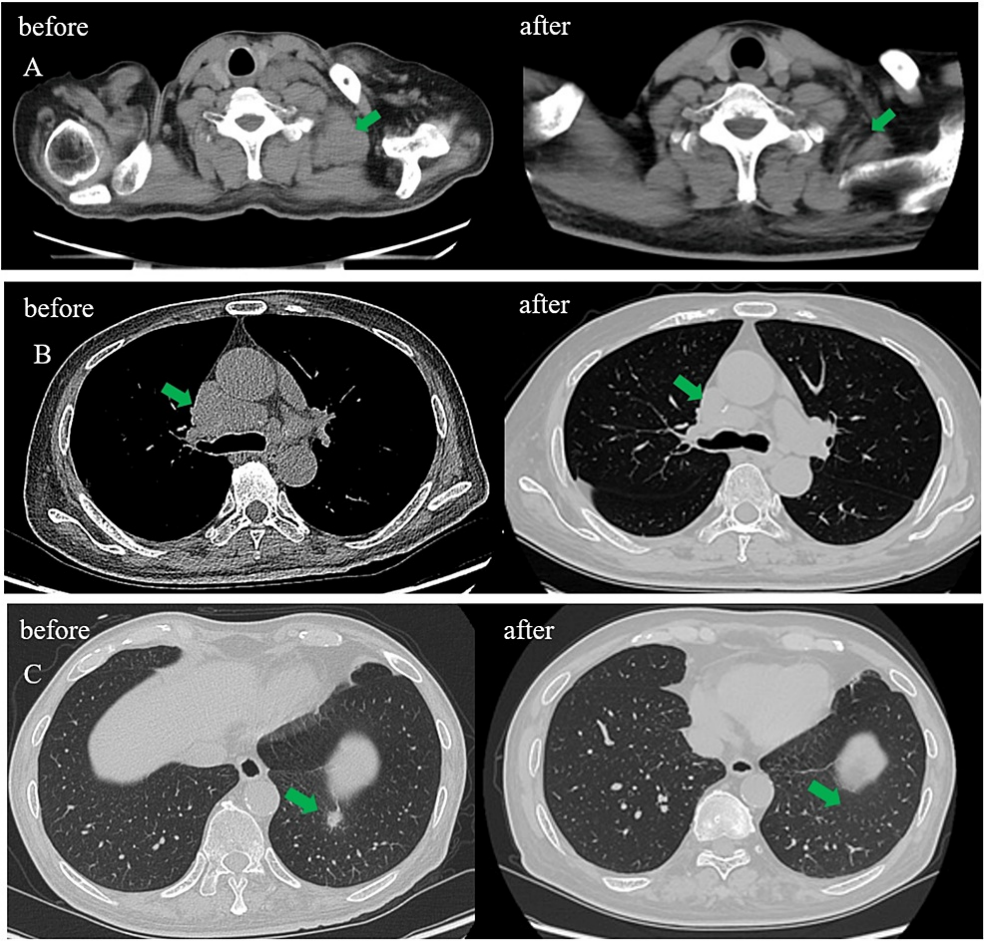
Panel A: Enlarged lymph nodes in the left cervical root on the chest CT scan (before), and lymph nodes diminished significantly after 18 weeks of prednisone acetate treatment (after); panel B: enlarged lymph nodes in the mediastinum on the chest CT scan (before), and lymph nodes diminished after 18 weeks of prednisone acetate treatment (after); panel C: enlarged nodules in the posterior basal segment of the lower lobe of the left lung on the chest CT scan (before), and nodules disappeared after 18 weeks of prednisone acetate treatment (after).
